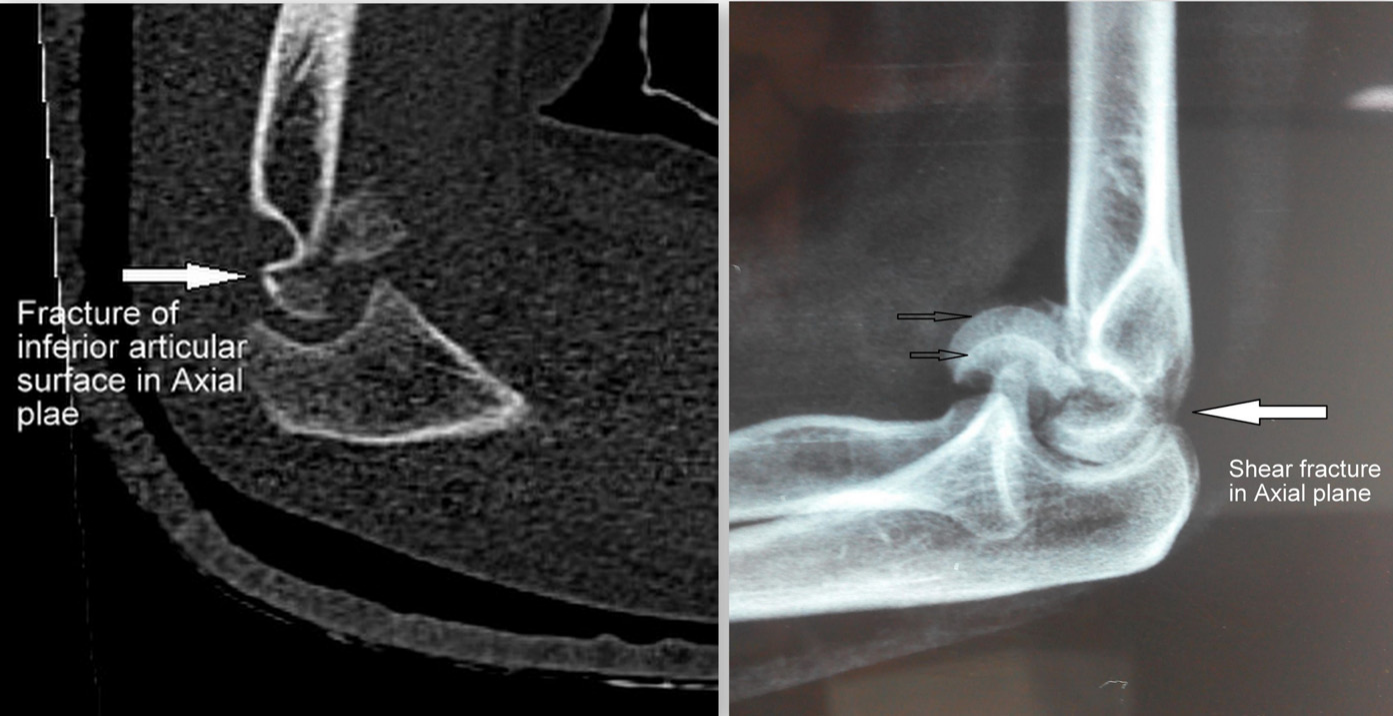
Sagittal section CT scan and X-ray images showing fracture of the inferior articular surface in axial plane.
